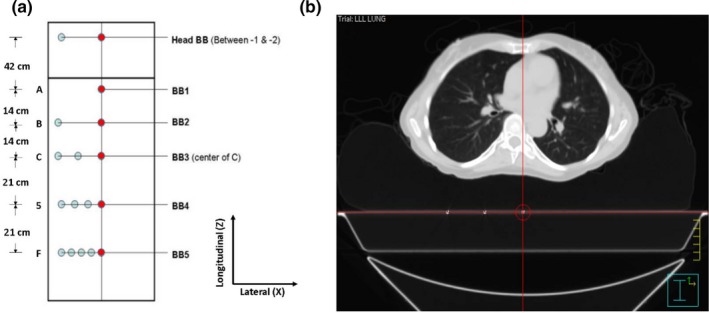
Ball bearings embedded couch design. (a) The arrangement of ball bearings (BBs) embedded in a CT couch‐top. The BBs on the midline are used as references for couch coordinate calculations; others are used to facilitate the reference marker identification. (b) An axial CT slice passes through a reference BB (BB_3_) selected as the laser coordinate system origin in the treatment planning system.
